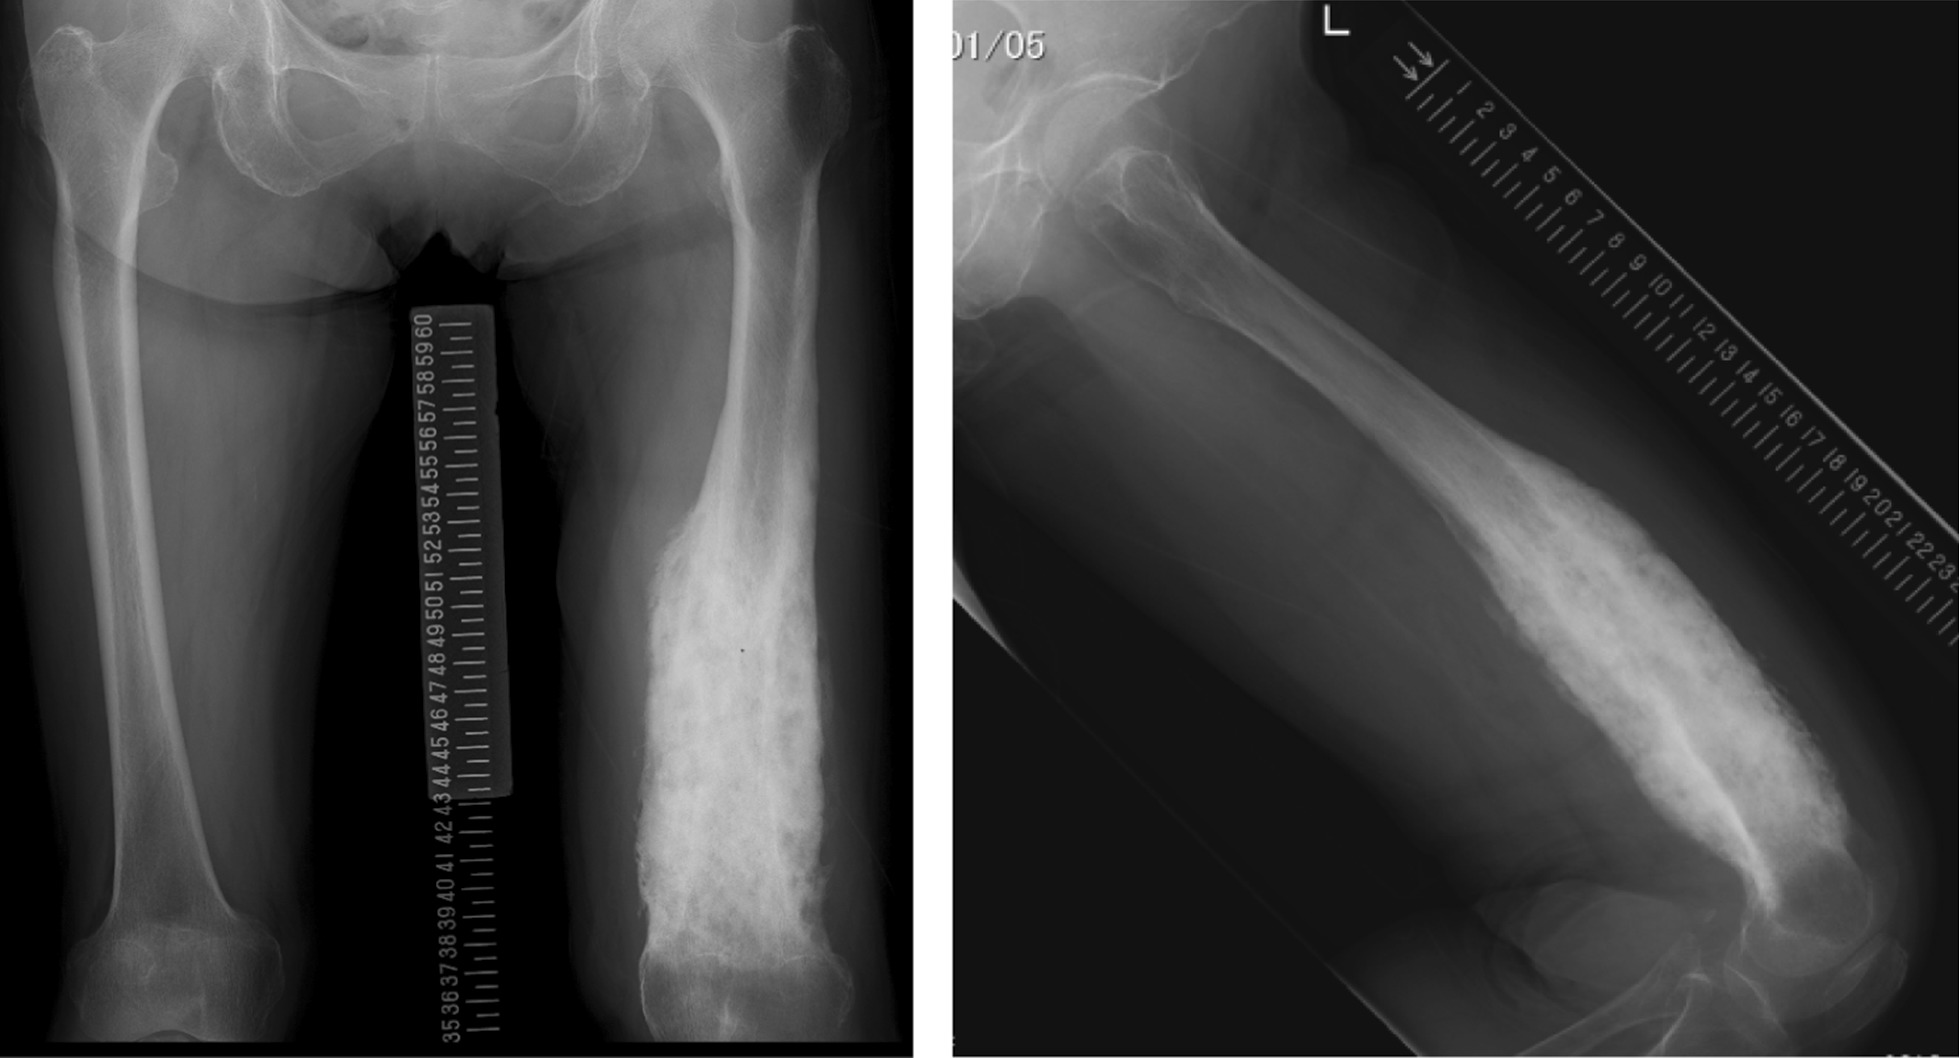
Radiograph of the left femur. Radiograph of the left femur showing an extensively osteogenic, thickened and sclerotic lesion from the mid-diaphysis to the distal femur. A dense, irregular margin was observed in the intramedullary and cortical lesions. The length of the sclerotic lesion is approximately 18 cm
